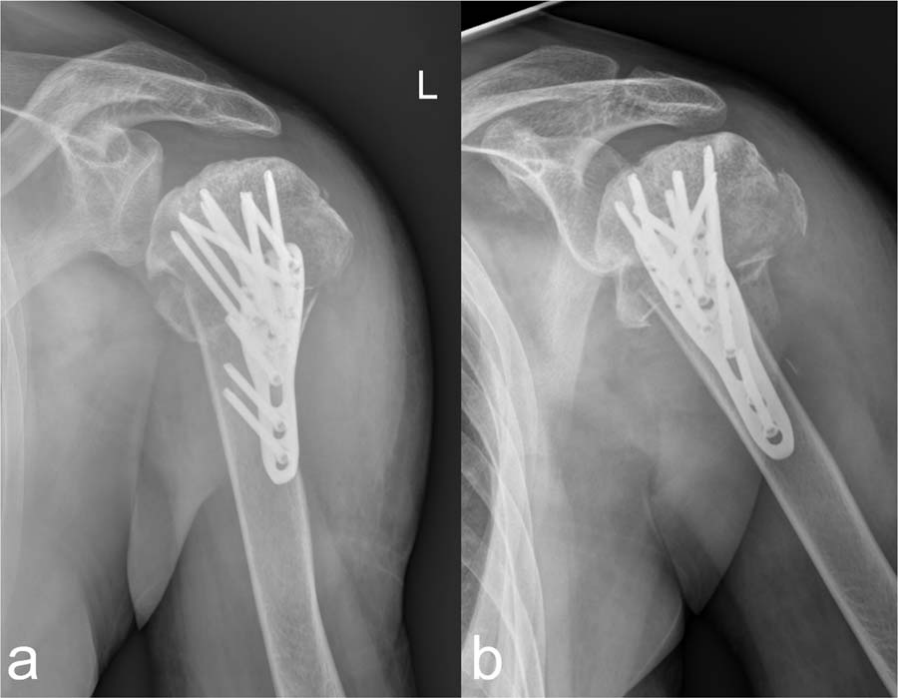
Treatment patterns of the subcapital humerus fracture (11C3.1). (a, b) The radiographs in two planes show extensive coverage of the fracture fragments with adequate stabilization of the multiple fragment fracture of the caput humeri, which was treated osteosynthetically using a PHILOS® plate and multiple screws.
